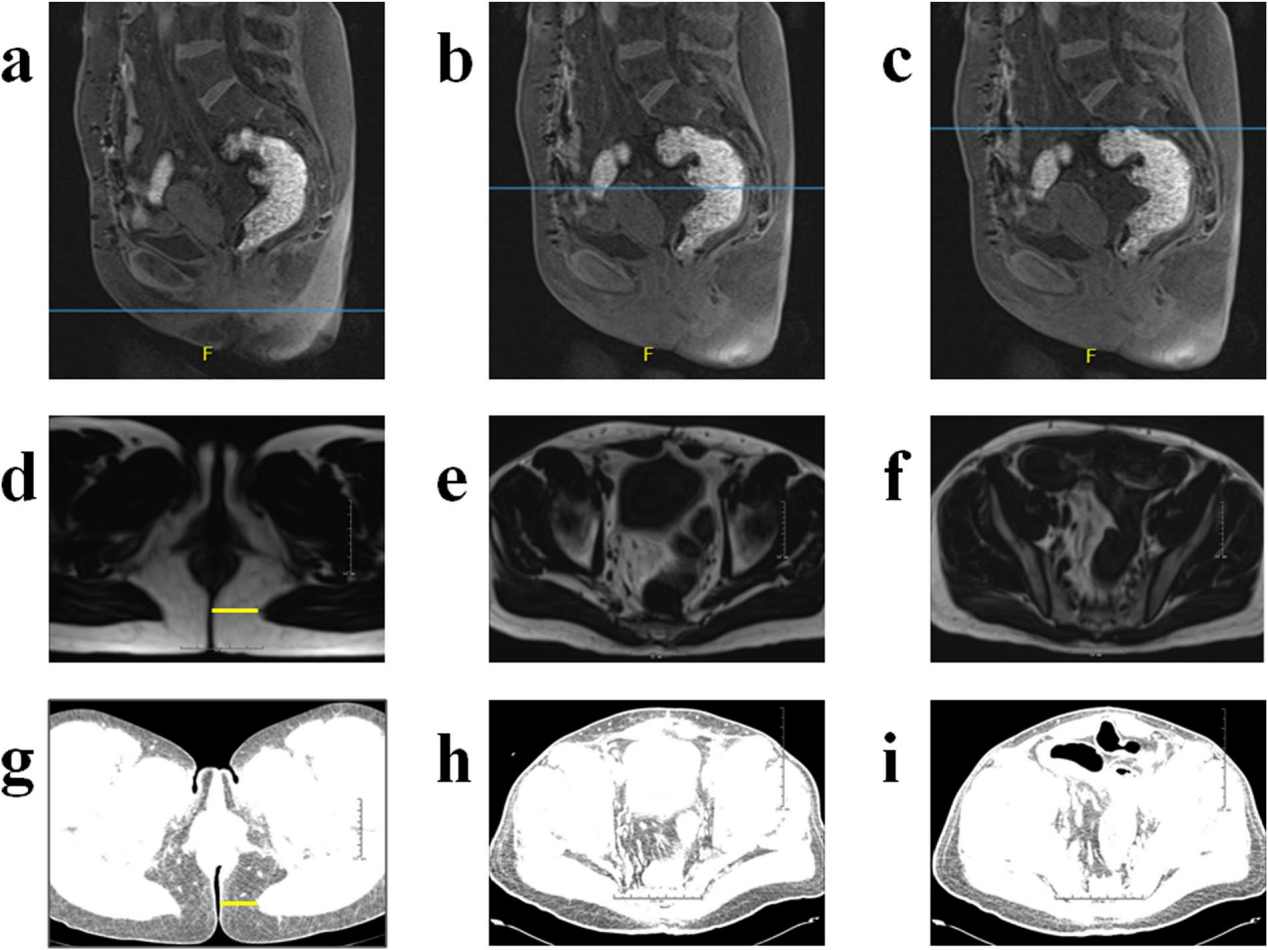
Sagittal MRI-DIXON-F images at the anal verge level (Level 1, (a)), the middle height level of pouch (Level 2, (b)) and the highest level of pouch (Level 3, (c)). Transverse cross-section MRI-DIXON-F images at Level 1 (d), Level 2 (e), Level 3 (f). Transverse cross-section CT images of the same patient at Level 1 (g), Level 2 (h), Level 3 (i).
